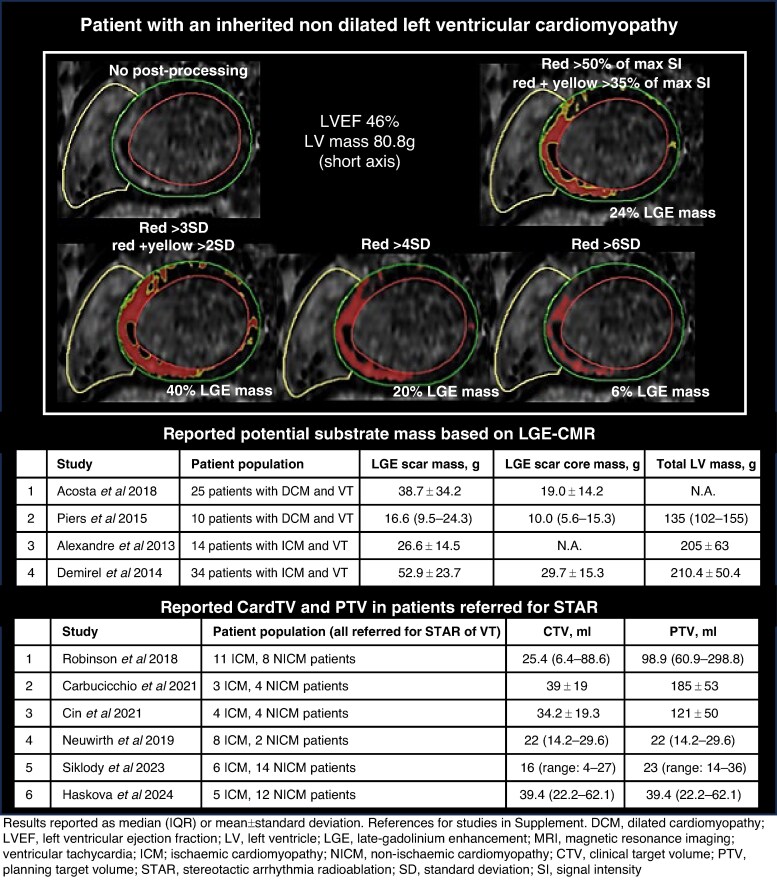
Variation in LGE based on threshold variation and reported CardTV-IMG based on LGE-MRI.
